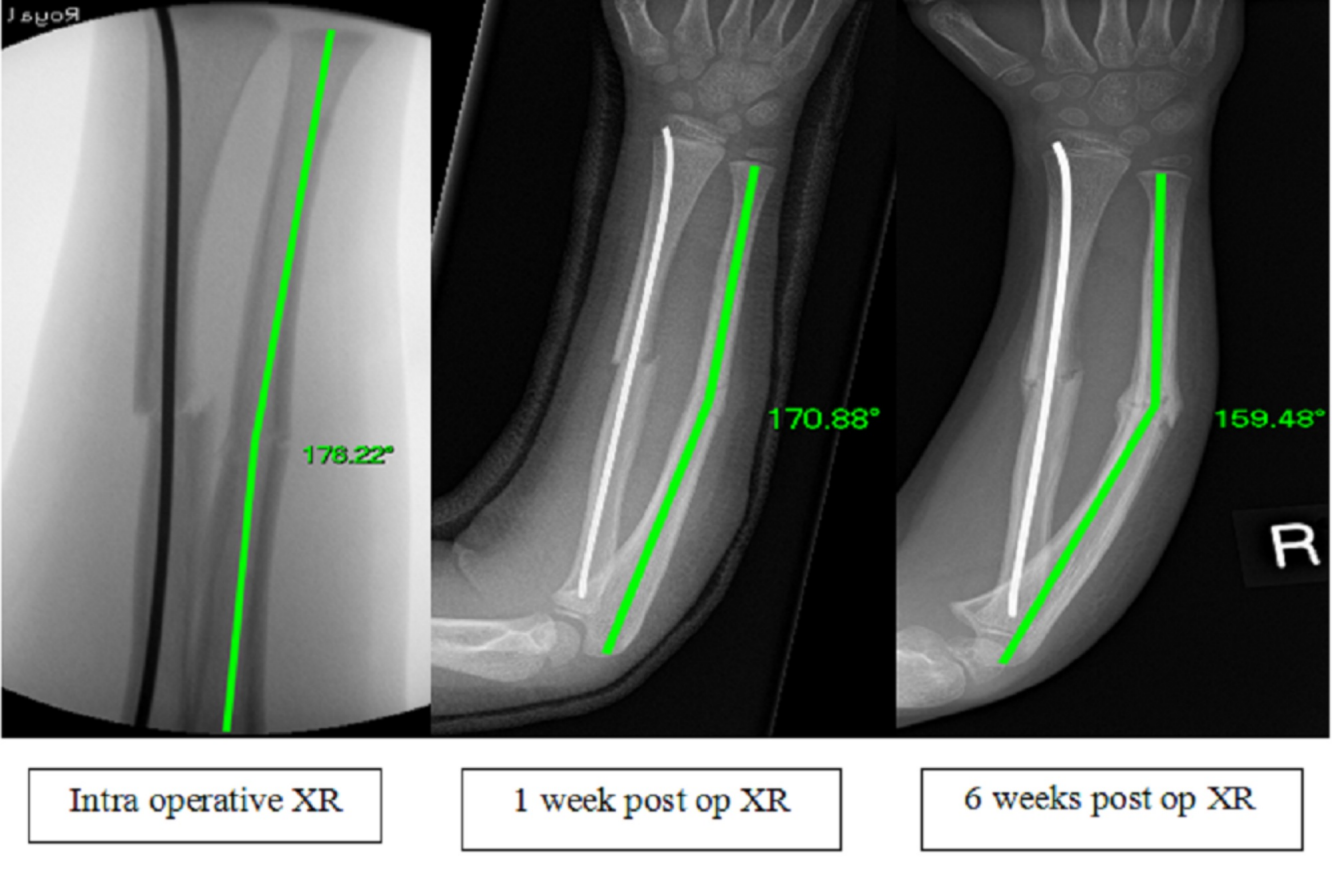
Intraoperative, 1 week and 6 week postoperative radiographs of S-EIN to radius with progressive deformity of ulna over time. Shown with on-screen angle measurement. S-EIN - Single elastic intramedullary nail
